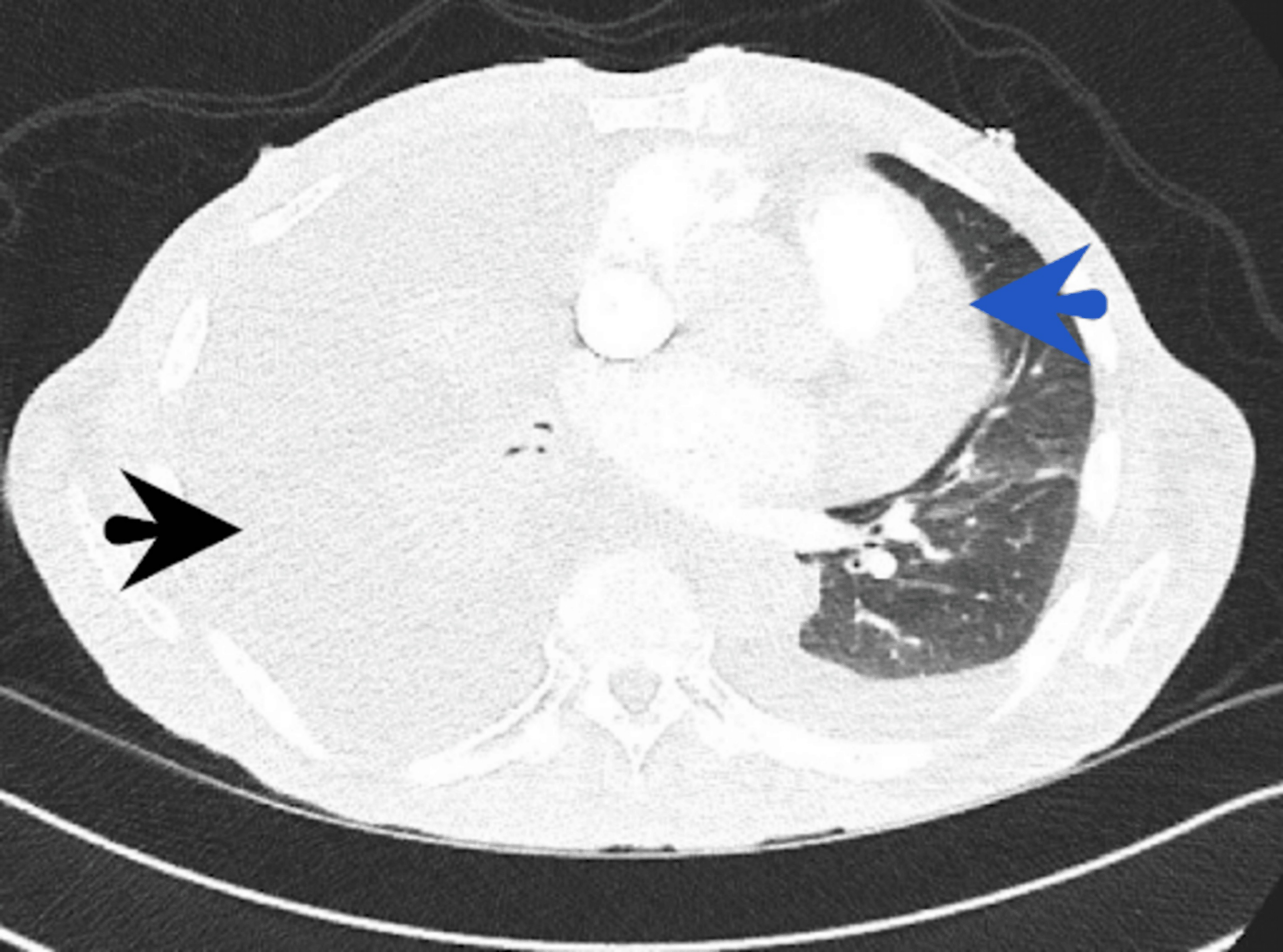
A CT scan of the chest confirmed a significant right-sided pleural effusion with a mediastinal shift to the left, along with features suggestive of tension hydrothorax (black arrow). A smaller left-sided pleural effusion was also noted (blue arrow).
